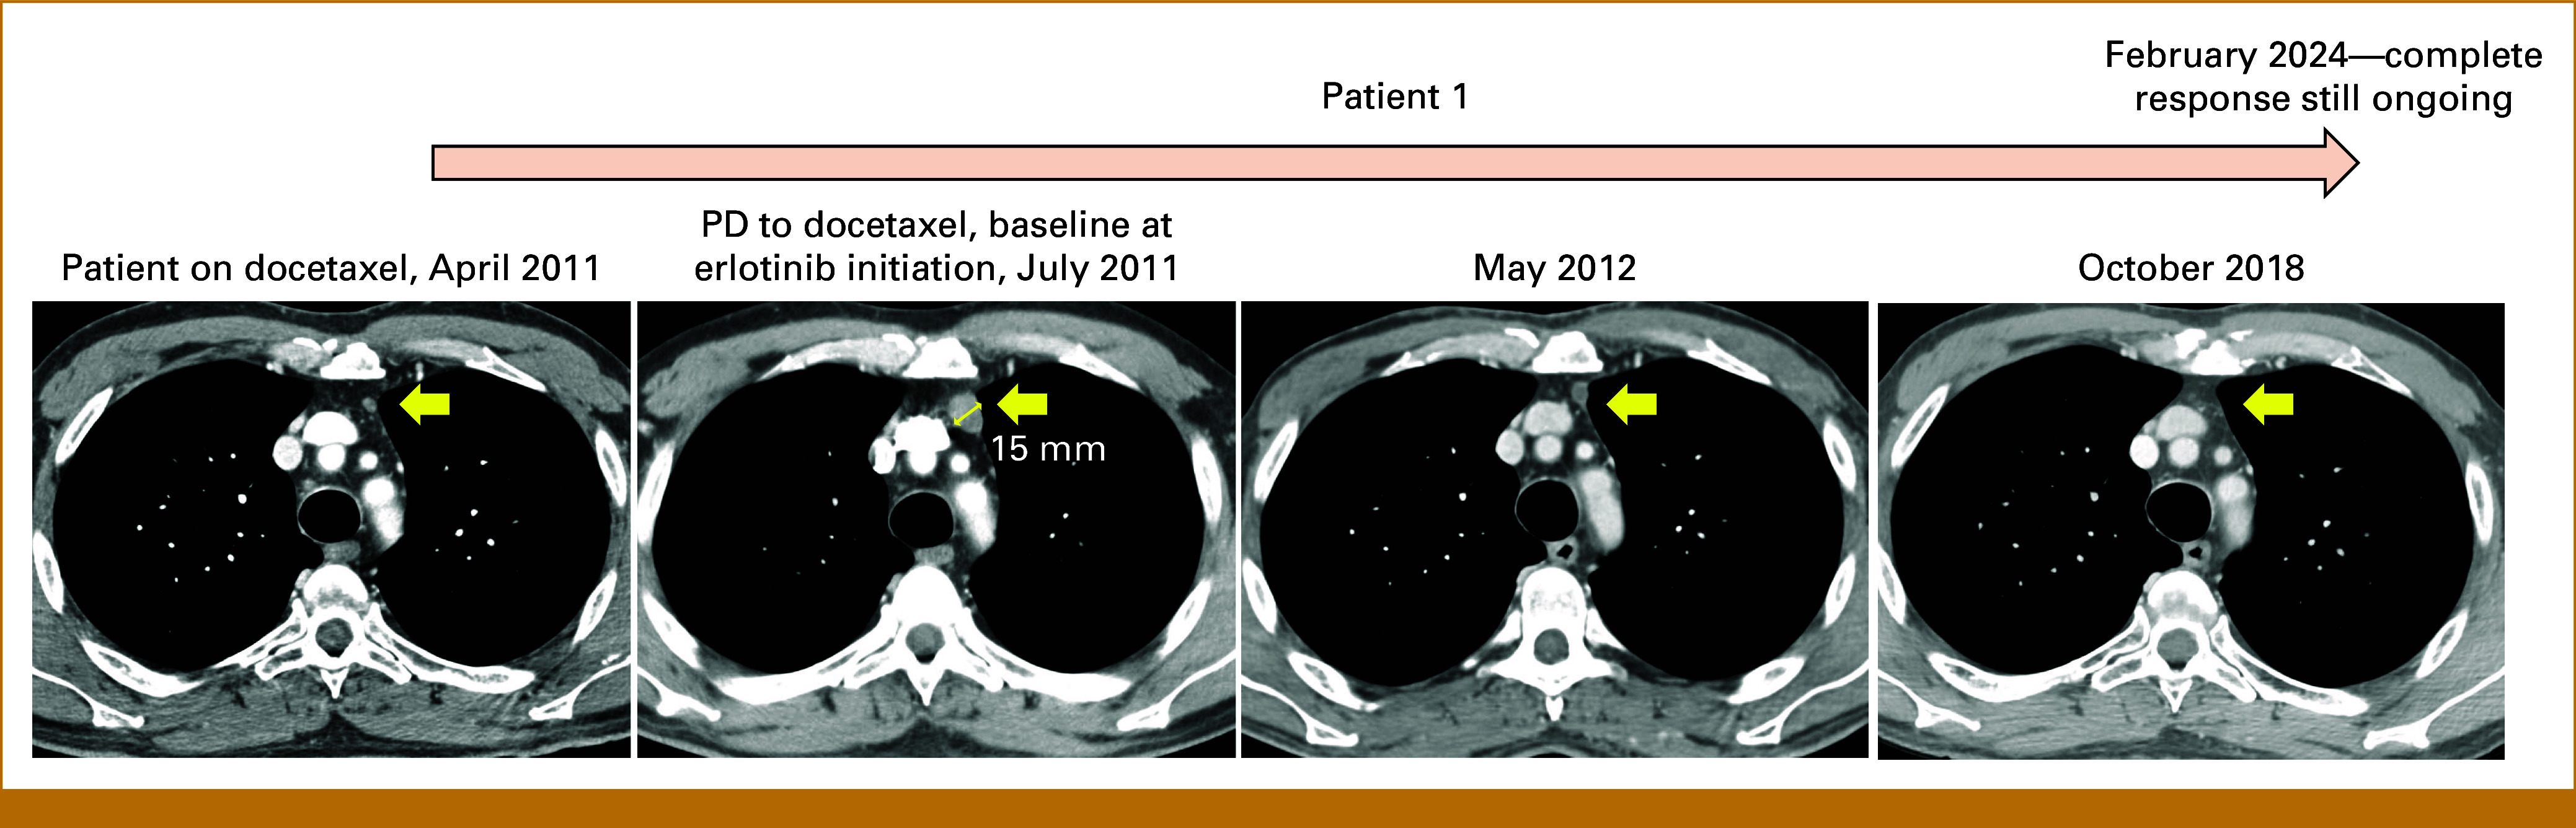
Radiographic assessment of erlotinib activity in patient 1. Computed tomography scans of patient 1 showing the tumor response to erlotinib after PD to docetaxel, with documented gradual shrinkage of a prevascular lymph node metastasis over time. PD, progressive disease.
